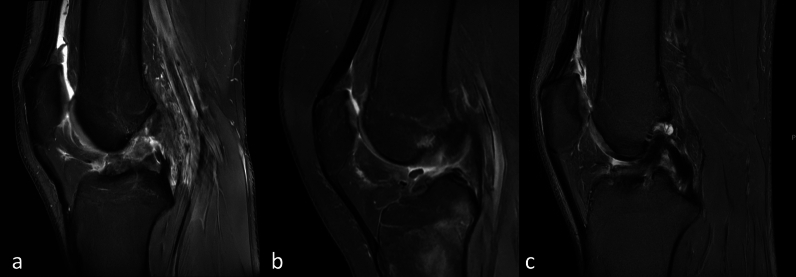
Sagittal proton density-weighted MR image of the knee demonstrating: a a distal rupture of the anterior cruciate ligament, b a mid-substance rupture of the anterior cruciate ligament, and c a proximal rupture of the anterior cruciate ligament
